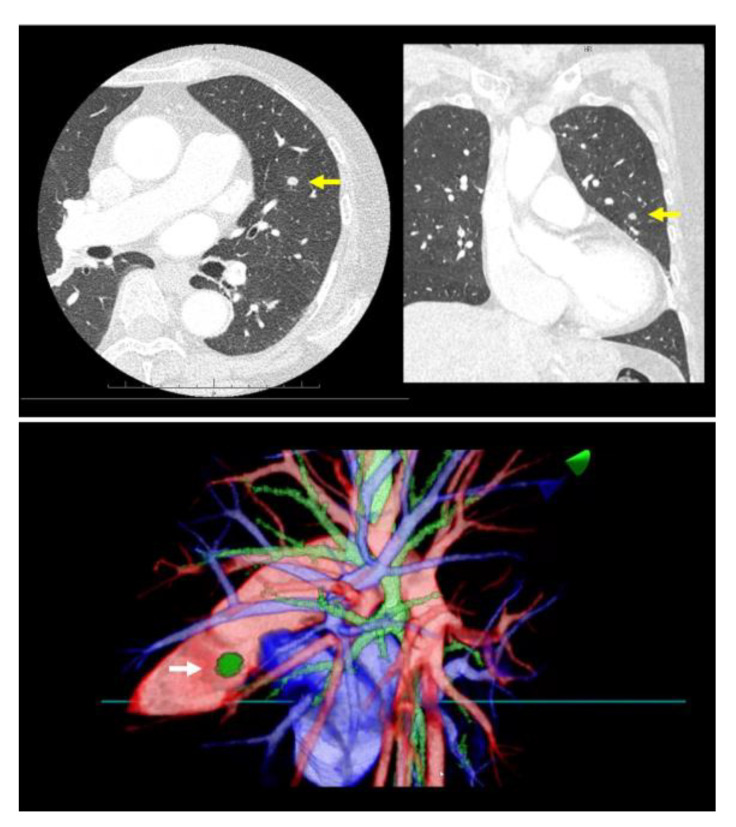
A virtual dynamic image is meticulously generated from patient-specific three-dimensional computed tomography (3D-CT) data, providing a detailed and interactive representation of the patient’s internal anatomy. The top row of images showcases a preoperative chest CT scan of a patient with a left lingular tumor (yellow arrow). The bottom row of images presents representative views of the 3D model with a left lingular tumor (white arrow) derived from the same CT data.
